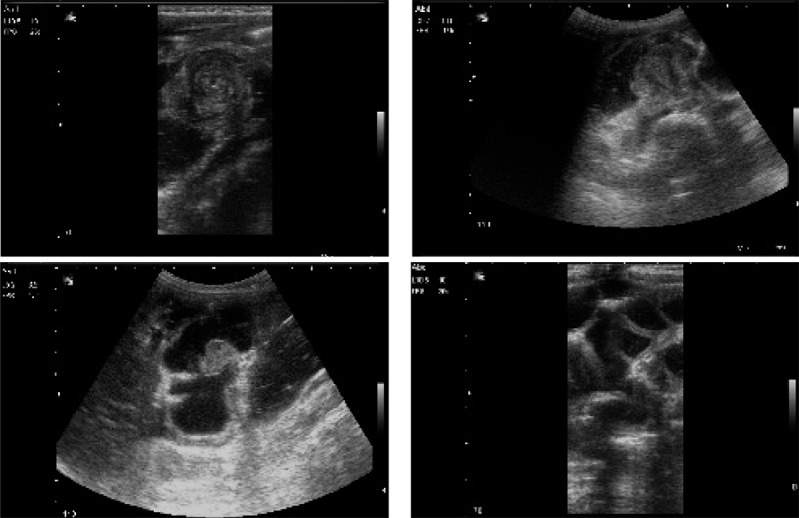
A 9-month-old boy presented with colicky abdominal pain from 36 hours ago. Sonography showed ileocolic intussusception with extension to the middle transverse colon. Successful reduction of intussusception by saline enema and sonographic guidance is noted.
